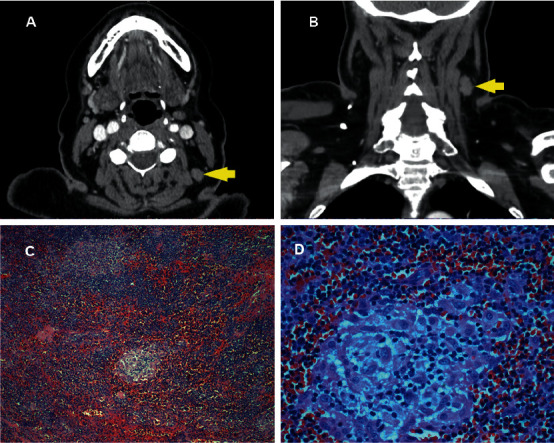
CT scan at transverse plane (a) and coronal plane (b) showing a 1.4 cm left level 5 A lymph node. No other adenopathy. No mass along the course of the aerodigestive tract. Cervical lymph node biopsy demonstrating lymph node with follicular hyperplasia, phagocytosis of nuclear debris, noncaseating epithelioid granuloma, and sinusoidal distension by plasma cells and immunoblasts (c) (x10 magnification). Higher magnification of the epithelioid granulomas (d) (x40 magnification).
